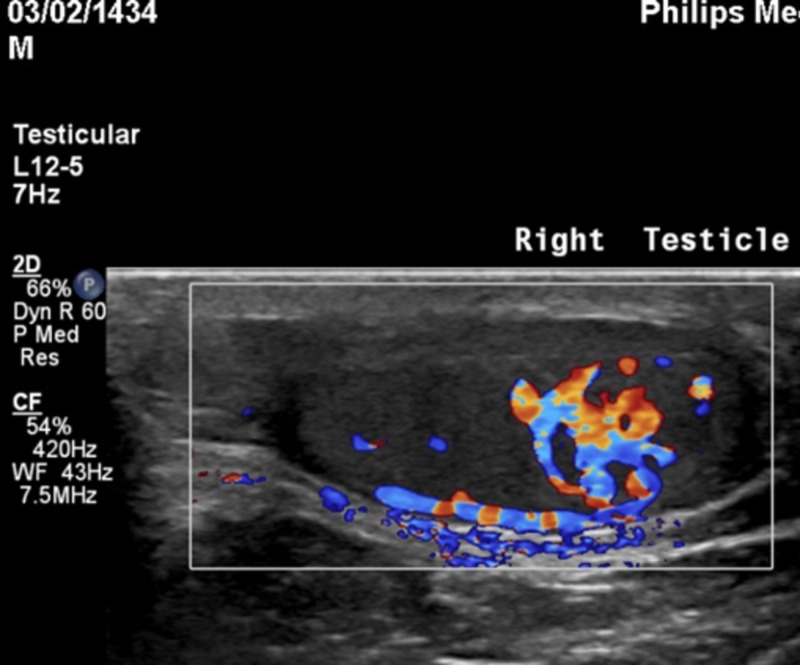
Testicular ultrasound shows right testicular mass
